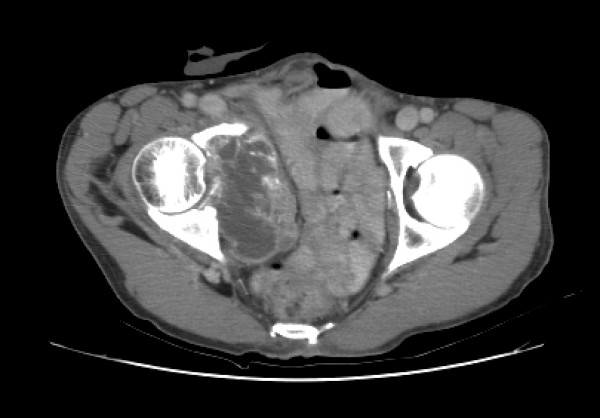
CT scan of pelvis showing a large locally destructive mass lesion. Showing a right sided large heterogeneous pelvic mass with an area of central necrosis with evidence of bone destruction (right acetabular invasion) and distal rectal involvement.
